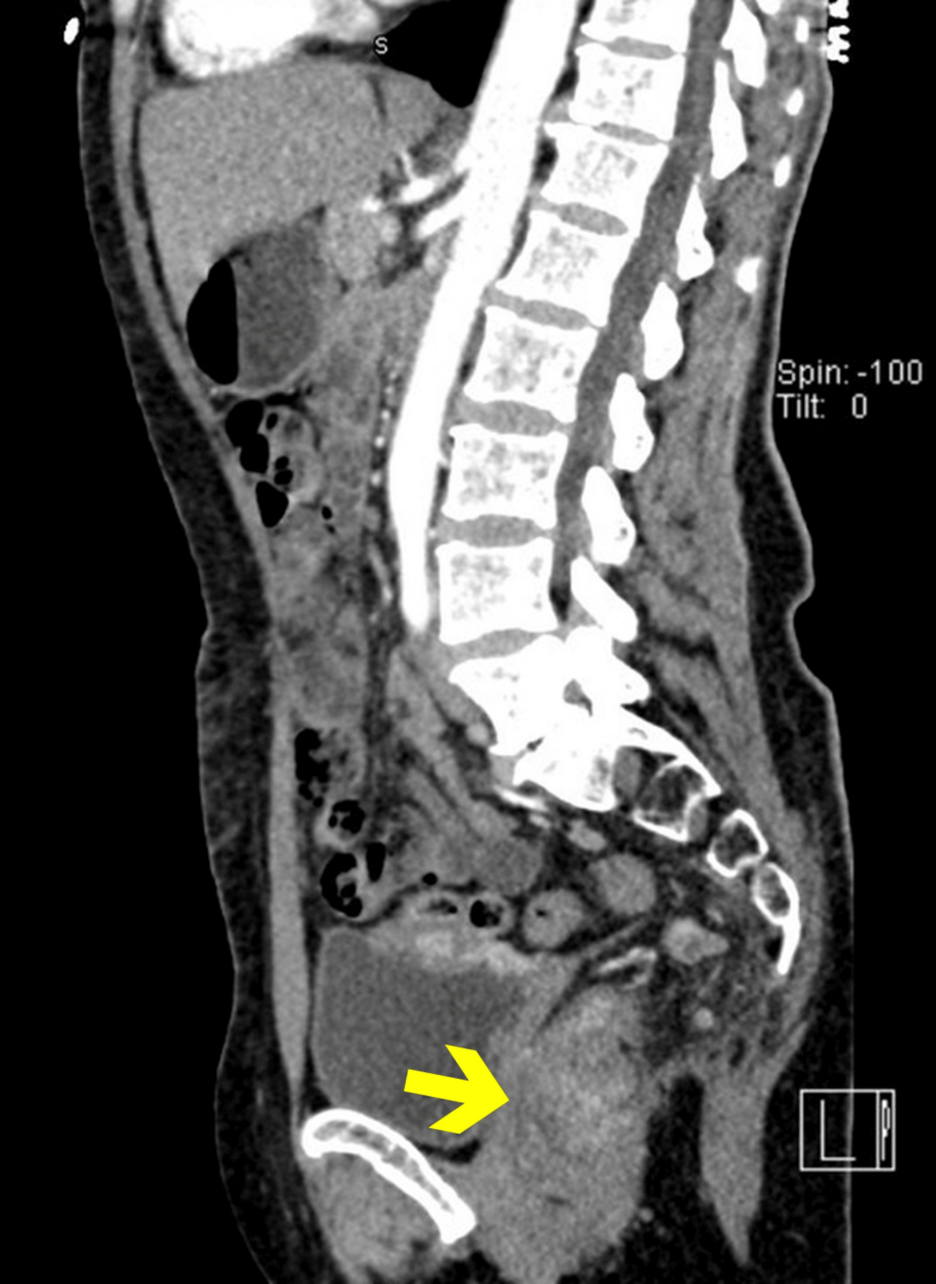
Sagittal CT image (contrast-enhanced). Sagittal CT reconstruction demonstrates an irregular mass centered in the rectum, with involvement of the rectal wall and partial obliteration of the fat planes with surrounding structures. The mass lies below the peritoneal reflection, without signs of bladder invasion or presacral bone destruction.
